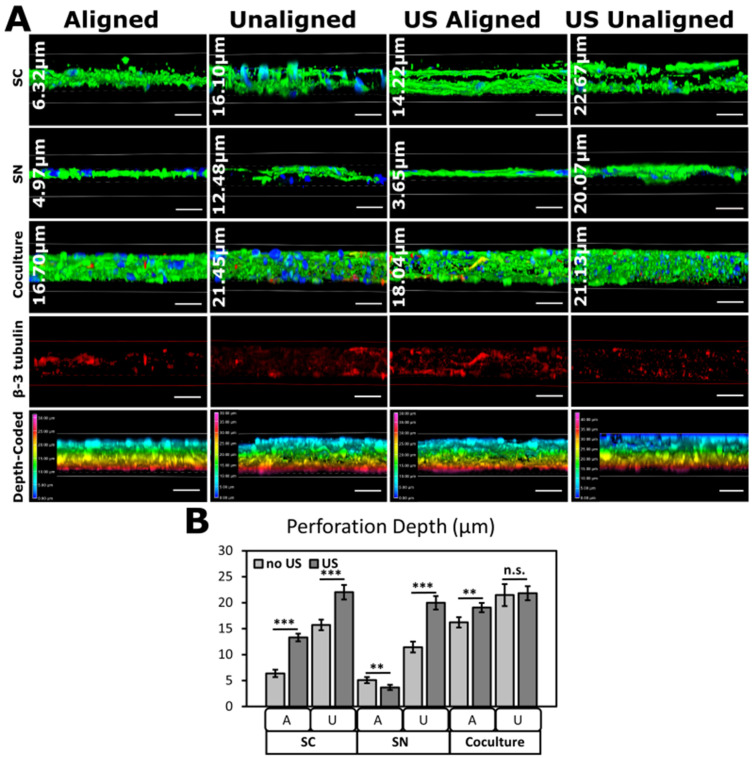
Perforation depth of SCs and SNs vertically into PVDF-TrFE. Ultrasound promoted perforation of SCs and SNs on unaligned scaffolds. (A) Representative immunofluorescence images of SCs and SNs seeded individually and in coculture on aligned and unaligned scaffolds with or without ultrasound treatment. Average perforation depth (µm) representative of a specific sample displayed on each image. Beta-III tubulin-only images show perforation of neurites in cocultures. Color-coded alpha-blended projections show perforation depth from XZ plane. Scale bars = 20 μm. (B) Quantified perforation depth of SCs and neurites with and without ultrasound. Culture times were 48 h for SCs, 72 h for SNs, and 5 days for SC-SN cocultures for stimulated and unstimulated scaffolds. Data reported as mean ± SD.  *p* ≤ 0.005; * *p* ≤ 0.0005. n.s.: no significance.
